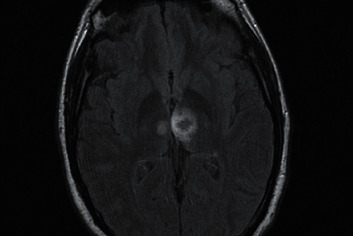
Magnetic resonance imaging where a bilateral acute thalamic infarction compatible with Percheron syndrome is observed appreciating hemorrhagic transformation in the left thalamus.
